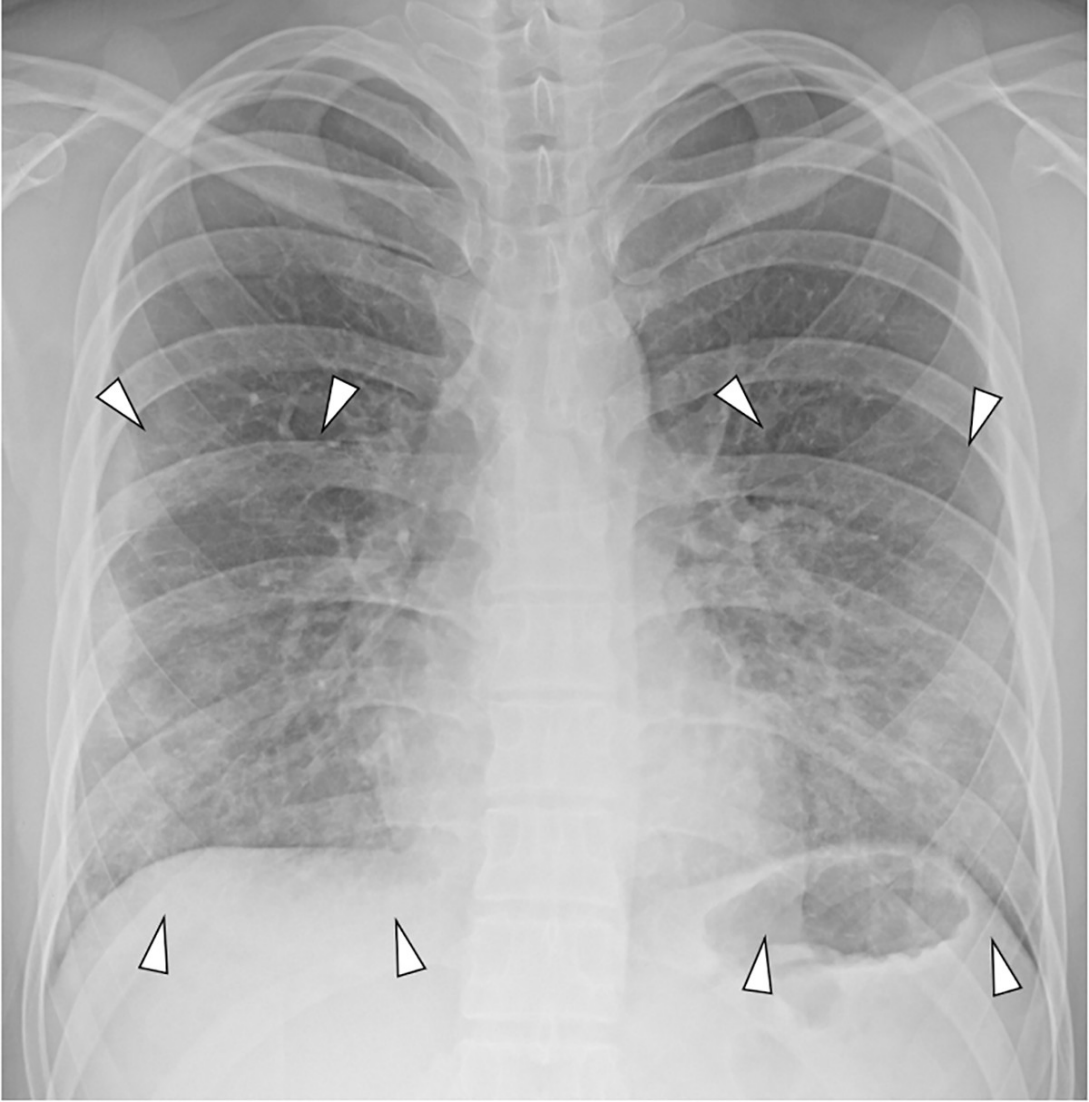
Chest radiograph (CXR) of a patient with mild COVID-19 disease. The initial CXR of a 29-year-old male patient with mild symptom. The patient reported rhinorrhea and chest pain at the time of CTC entry. However, the initial CXR showed diffusely increased opacities in both the mid- to lower-lung zones (arrowheads). Dyspnea was aggravated the following day, and the patient was transferred to a hospital.
